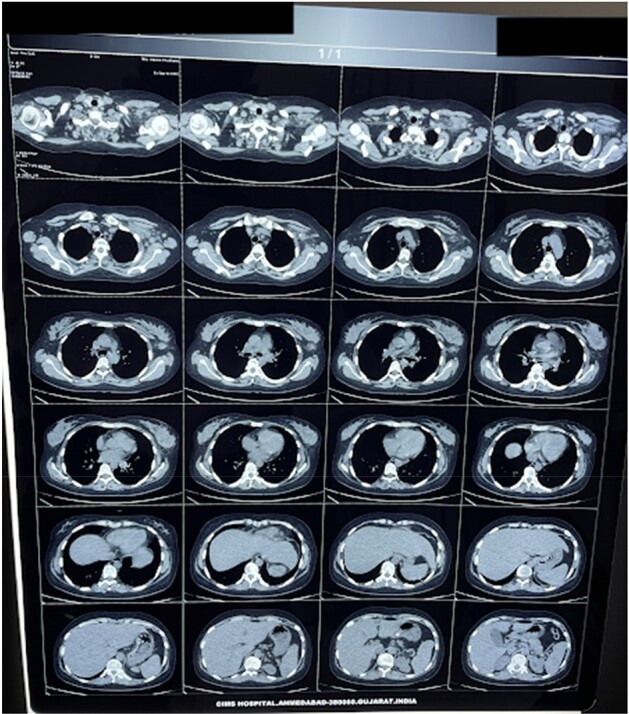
HRCT THORAX shows multiple, discrete, randomly distributed nodules in both the lungs, multiple enlarged lymph nodes in left axilla and in left anterior chest wall, some of which are confluent in nature and two calcifications in one of the lymph node.
